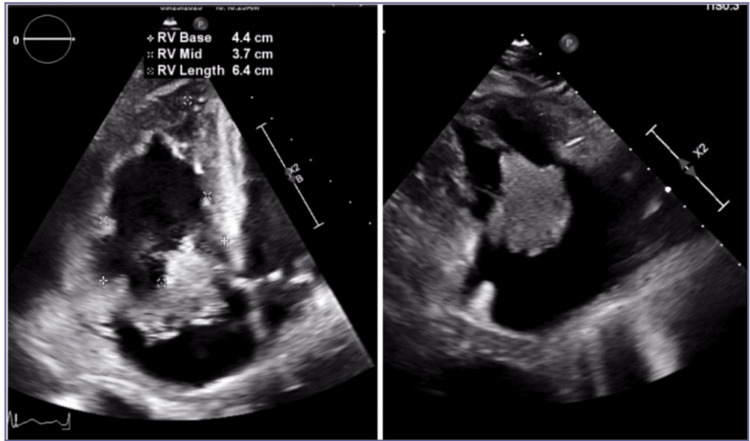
Transesophageal echocardiography demonstrating a 3 × 3 × 3 cm lobulated right atrial mass with a narrow pedunculated attachment just superior to the anterior tricuspid annulus. The mass prolapses through the tricuspid valve into the right ventricle during diastole, resulting in severe tricuspid regurgitation. Imaging features suggest a high likelihood of infective vegetation rather than thrombus, guiding multidisciplinary management decisions.
